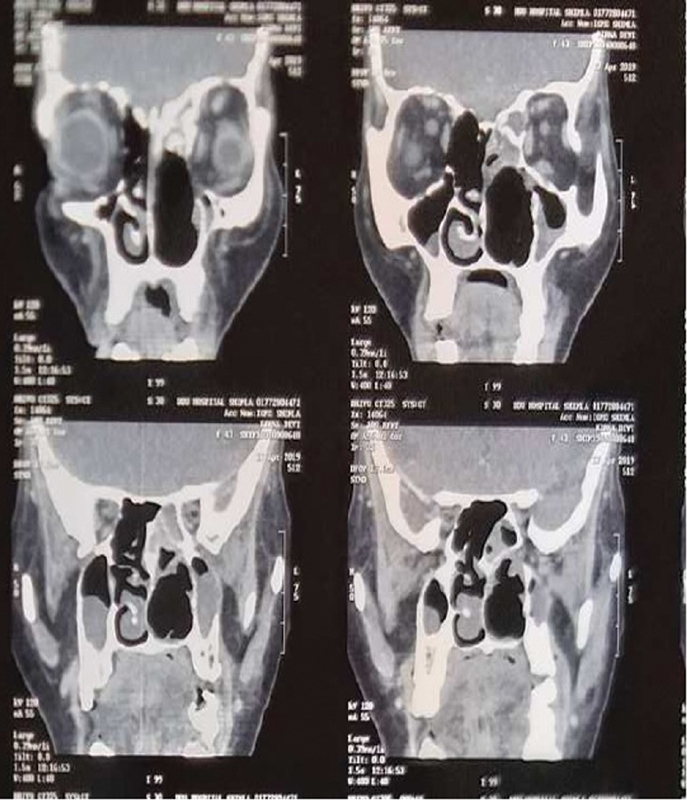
Postoperative computed tomography scan with no evidence of tumor grossly.
